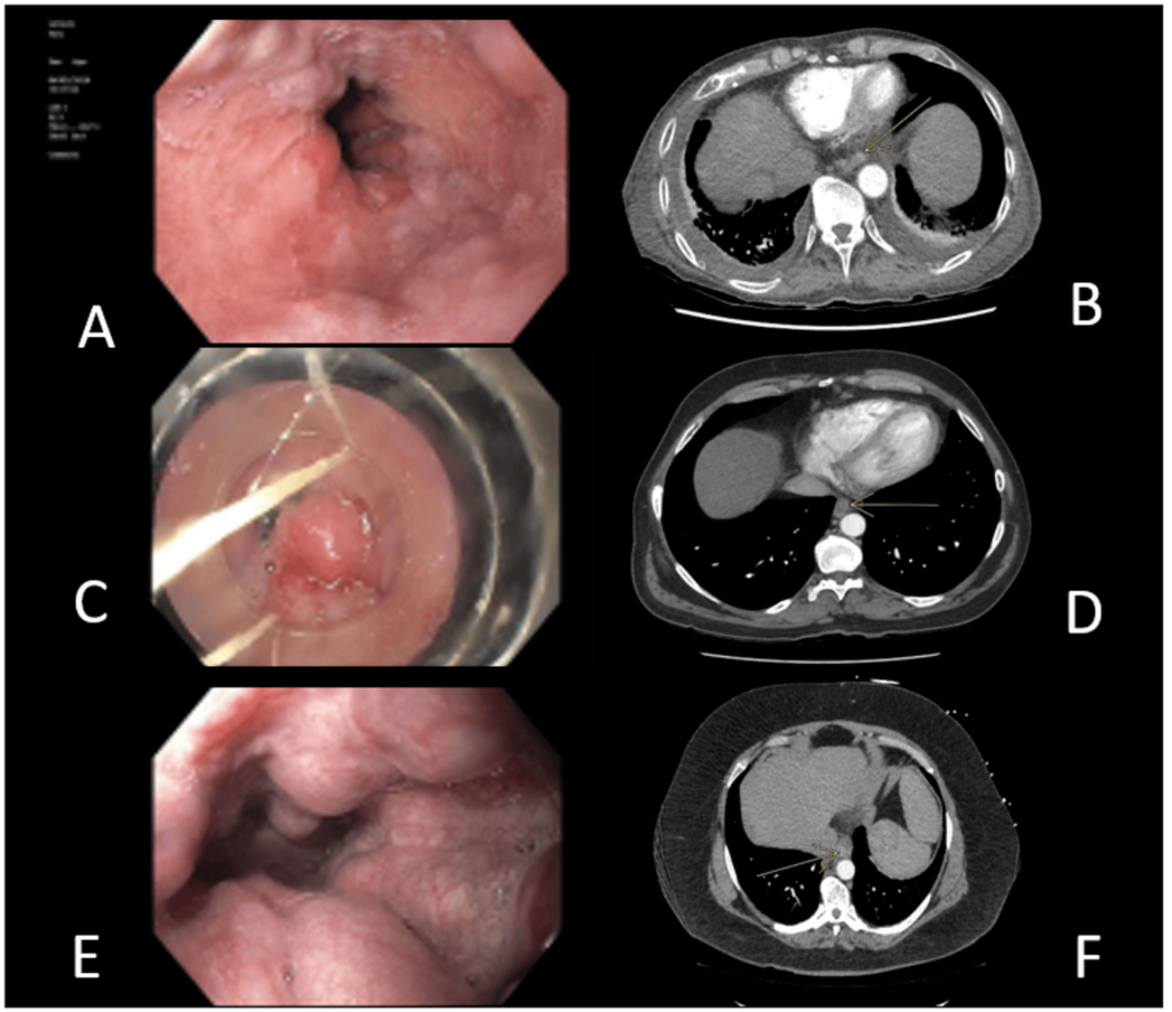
Oesophageal varices located in the lower 1/3 of oesophagus OGD: oesophagogastroduodenoscopy; CT: computed tomography; OV: oesophageal varices OGD (A) and corresponding arterial phase contrast-enhanced CT (B) of a patient with grade I OV  OGD (C) and corresponding arterial phase contrast enhanced CT (D) of a patient with grade II OV OGD (E) and arterial phase contrast enhanced CT (F) of a patient with grade III OV
